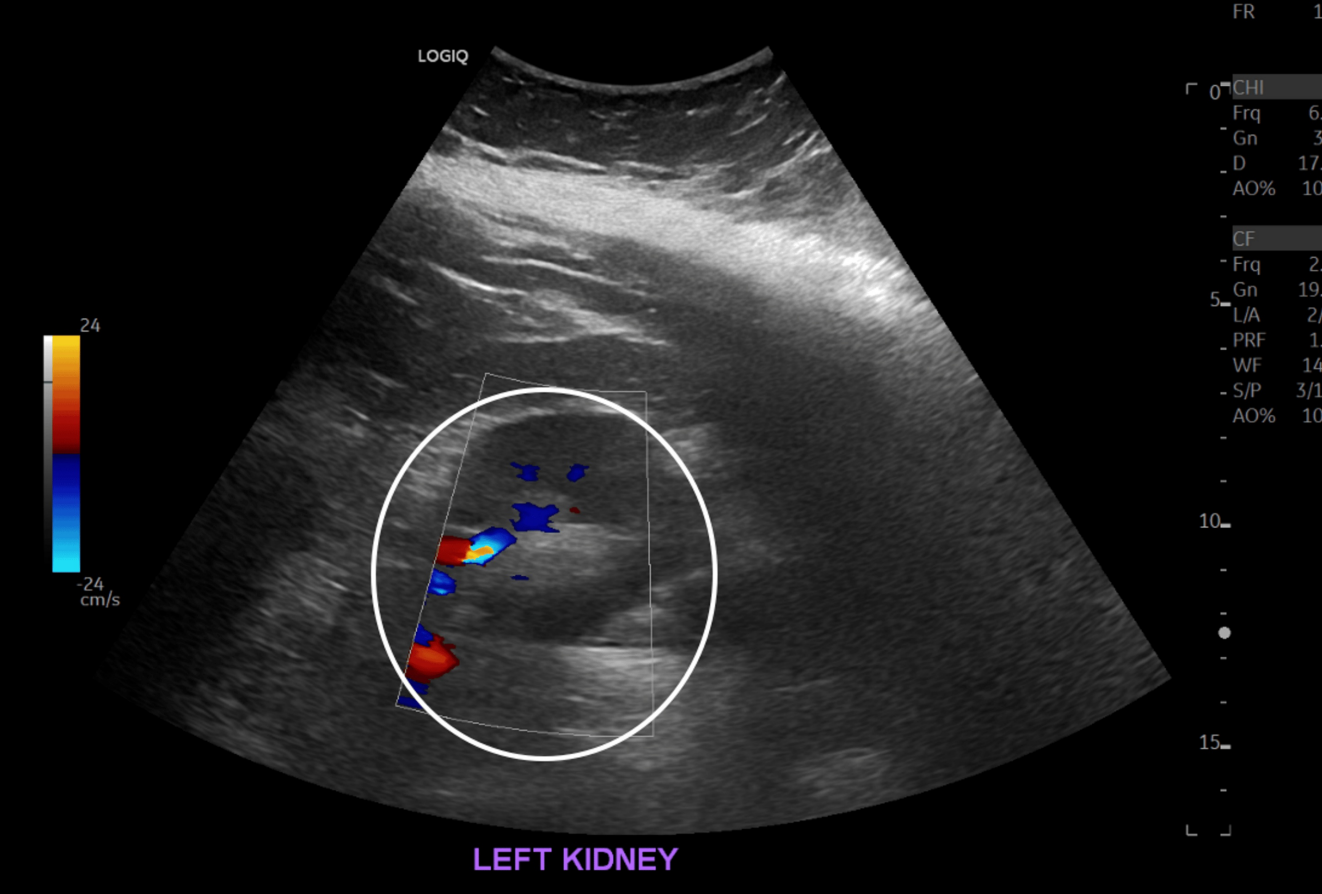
Left kidney ultrasound demonstrates increased echogenicity suggestive of ongoing glomerular inflammation and chronic fibrotic changes characteristic of antineutrophil cytoplasmic antibody (ANCA)-associated vasculitis.
